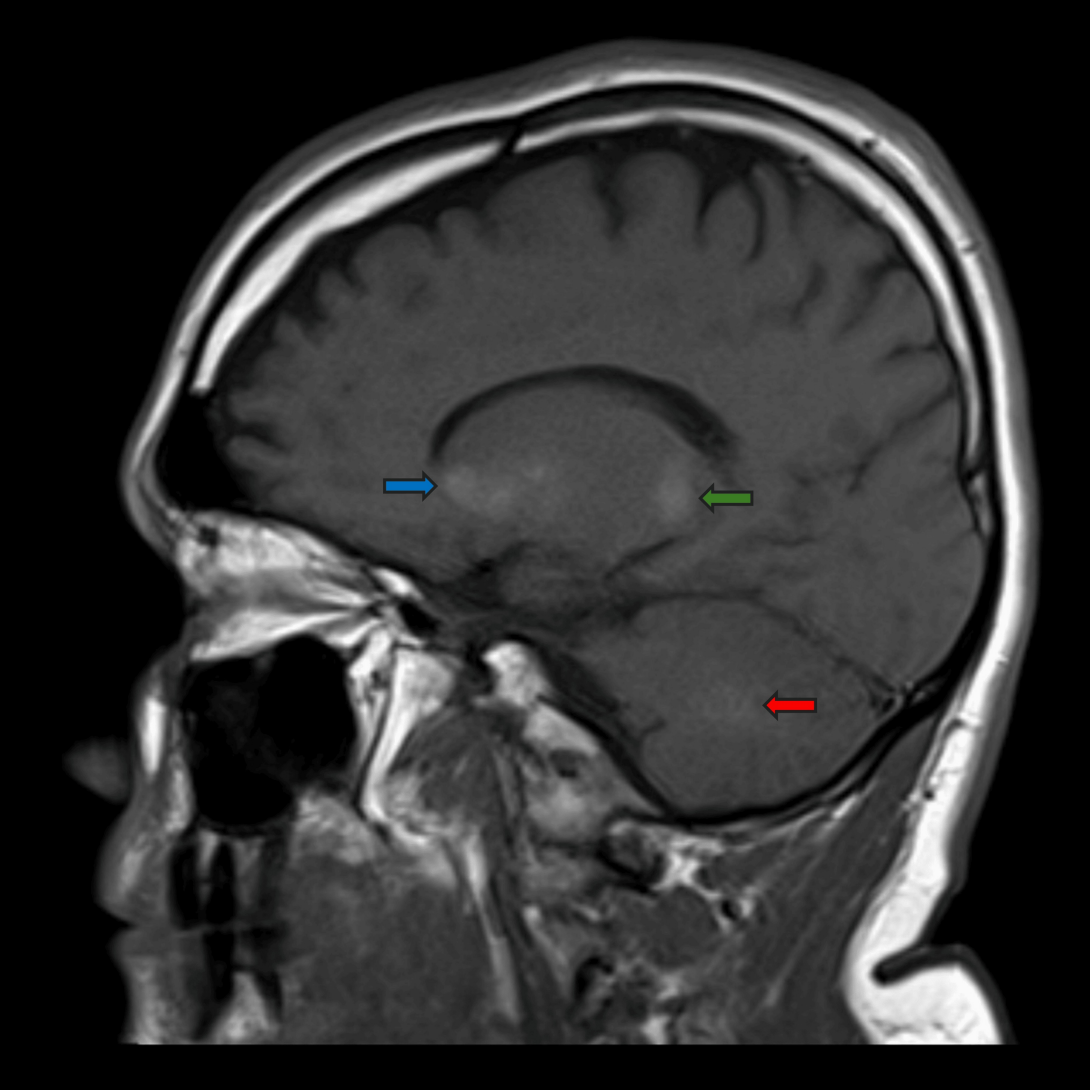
Non-contrast MRI of the head, sagittal section ​​​The sagittal T1-weighted image confirms the widespread mineralization seen on CT, visible as areas of hyperintensity (bright signal). Blue arrow: calcification within the basal ganglia; green arrow: calcification within the thalamus; red arrow: calcification within the cerebellum CT: computed tomography; MRI: magnetic resonance imaging
